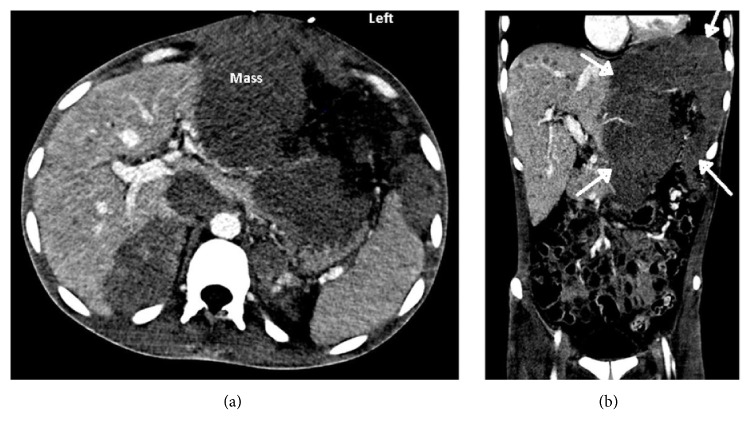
(a) Computed tomography with contrast of abdomen showing 16.3 cm × 14.1 cm × 20 cm hepatic mass (axial view). (b) Computed tomography with contrast of abdomen showing mass (arrows) involving the left hepatic lobe (coronal view).
